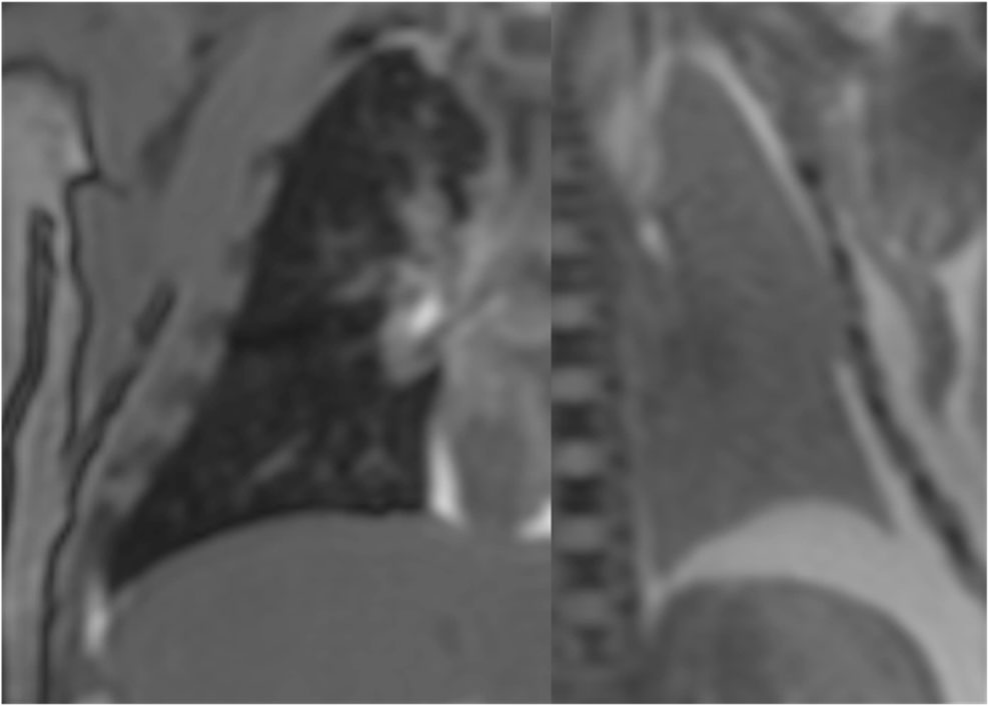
PMMR for lung aeration. Signal intensity differences in the lungs on PMMR may be used to differentiate between a baby who has breathed (dark airways and lungs on coronal T2-weighted PMMR image in a 2 week old baby; a) versus one that has not (light lungs in a 30 week gestation fetus with no signs of life at delivery; b). Reproduced with permission from [54] under Open Access agreement
